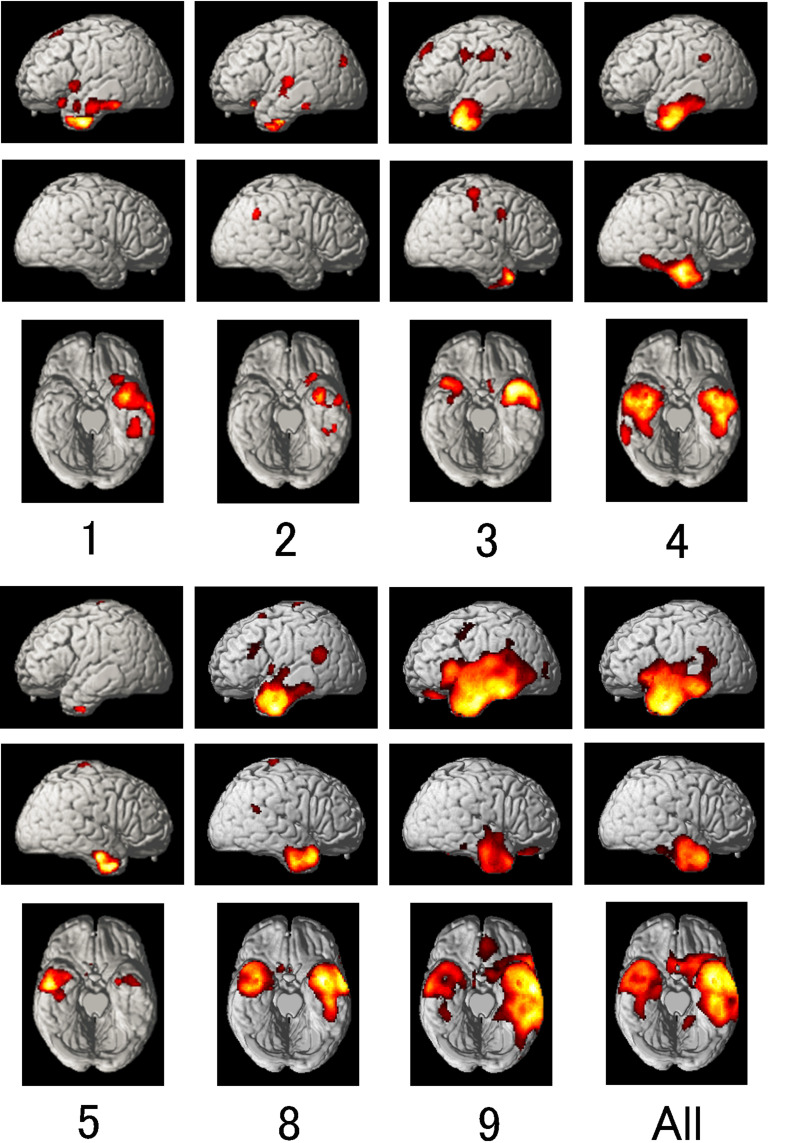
ECD-SPECT images of the 7 patients individually and altogether. Number corresponds to the patient number in Table 1. Each patient’s images were compared with those of normal subjects of the same generation and sex, using easy Z-score Imaging System (eZIS) and Statistical Parametric Mapping version 2 (SPM2). Areas of hypoperfusion (uncorrected *p* < 0.001 on *t*-test for individual analyses and analysis of covariance for all patients) are presented with a red-to-yellow gradient representing increasing z-scores. Temporal lobe hypoperfusion was left-predominant (Patients 1–3, 8, and 9), right-predominant (Patient 5), and left-right nearly equal (Patient 4). Averaged surface images revealed left-predominant hypoperfusion, involving the left posterior inferior frontal gyrus and anterior temporal lobe extending to the mid-fusiform gyrus. Areas with local maximum blood flow reduction in the temporal lobe were the left anterior and middle inferior temporal gyrus and mid-fusiform gyrus [Brodmann area (BA) 20; (–40, –4, –40), (–42, –24, –28), (–46, –42, –26)] and the right anterior temporal pole and middle and inferior temporal gyrus [BA 21; (42, 12, –32), (56, –8, –22), (42, –8, –34)] on the Montreal Neurological Institute (MNI) coordinate.
